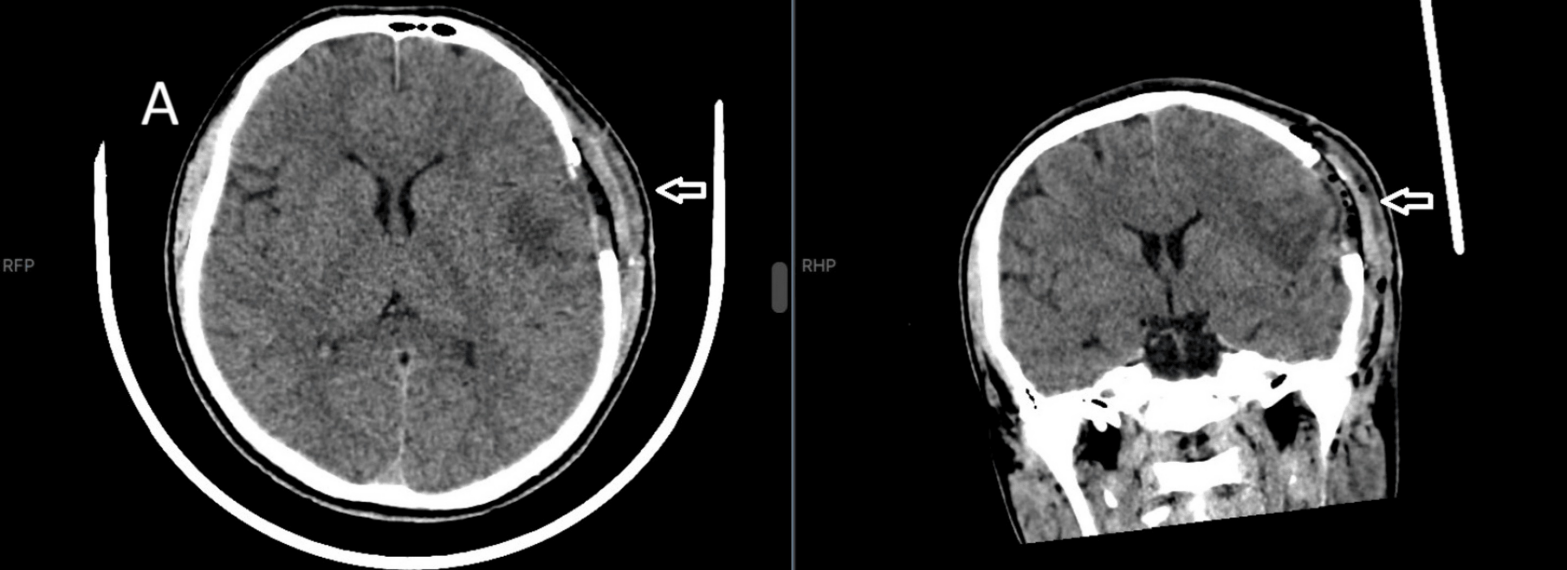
Postoperative non-contrast CT scan. (A) Axial view demonstrating post-surgical changes following left frontotemporal craniectomy for intra-axial lesion resection. The arrow indicates the left frontotemporal surgical cavity, measuring approximately 3.7 × 3.3 cm, with residual hemorrhagic content and perilesional white matter edema. (B) Coronal view; the arrow points to the left frontotemporal surgical cavity, showing surrounding vasogenic edema and a thin layer of subarachnoid hemorrhage. An extradural hydroaeric collection is noted adjacent to the craniectomy defect, likely containing hemostatic material.
